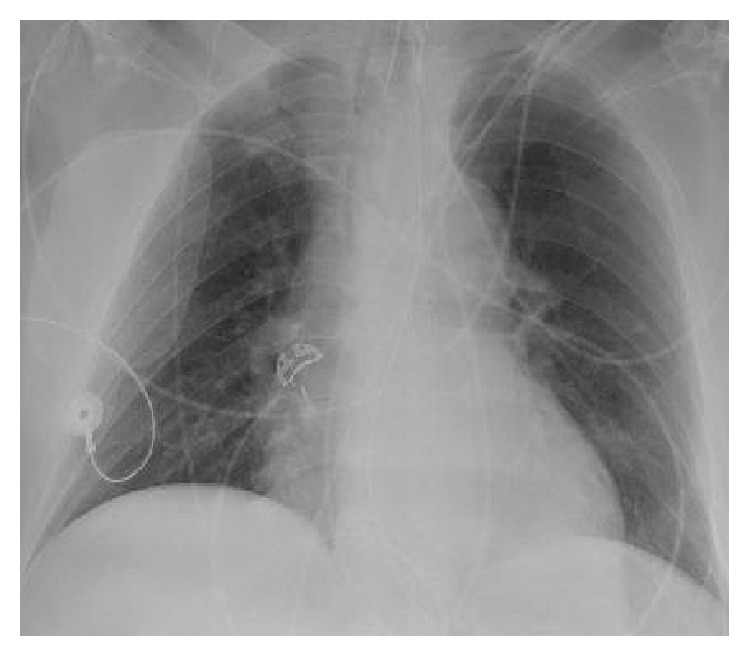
Chest X-ray. Inflated lungs with right upper lobe opacity and a high endotracheal tube position caused by a foreign body in the central airway.
